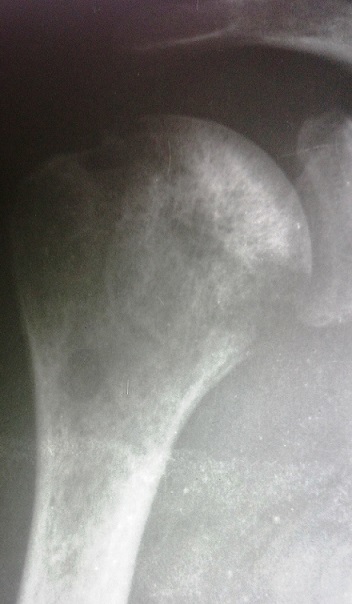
Radiographie de l’épaule droite de face aspect hétérogène de la tête humérale, avec des géodes
